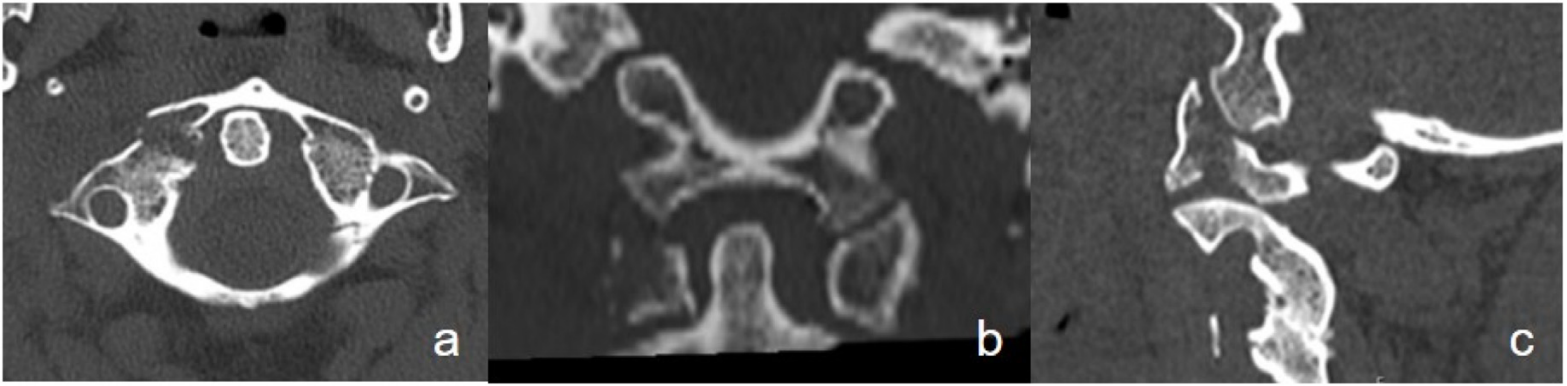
Preop CT scan of C1. a) axial, b) coronar, c) saggital plane.
